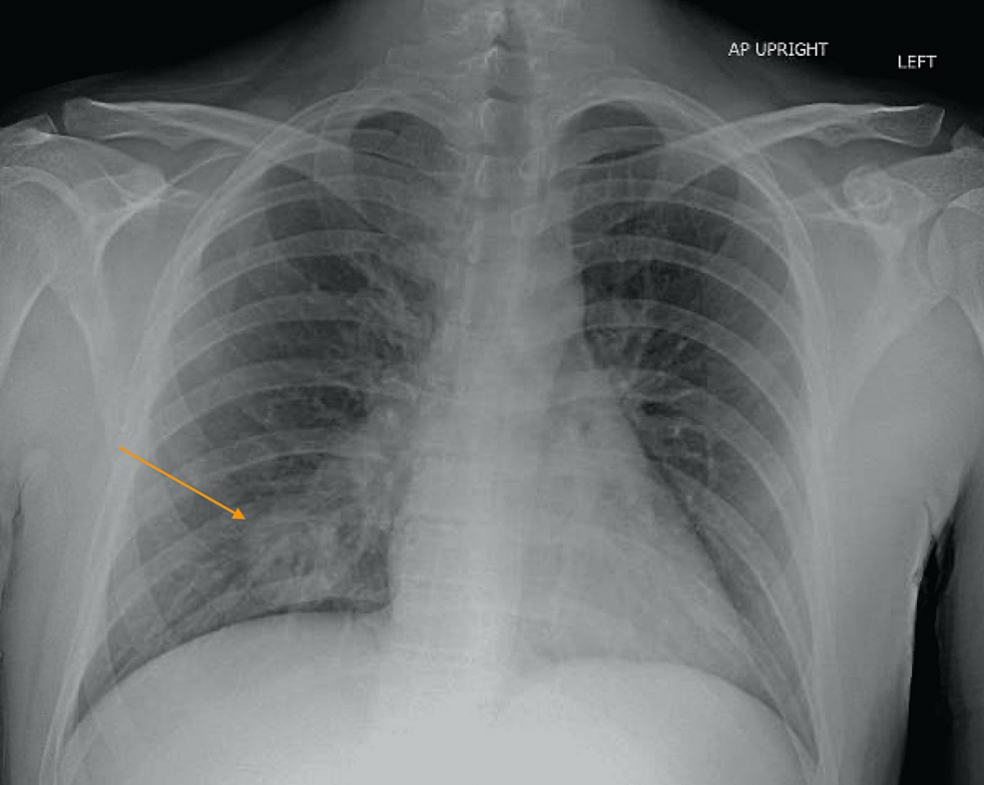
Chest x-ray showing the presence of a mass-like opacity in the medial right lung base measuring 4.1 x 4.0 cm with potential cavitation, as indicated by the arrow.
